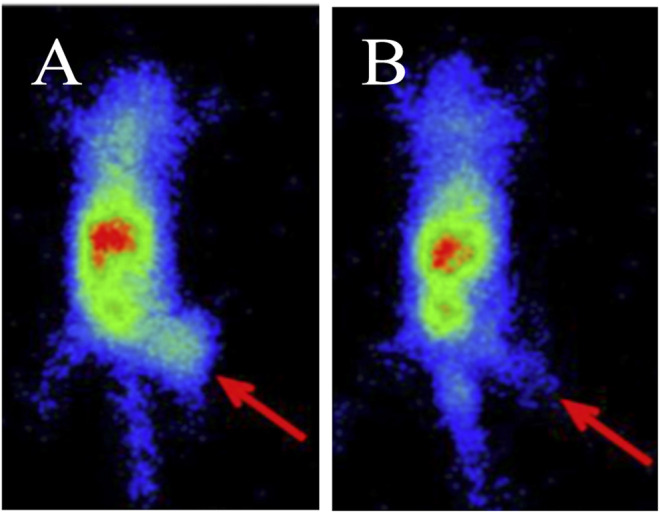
SPECT Imaging of MDM2 expression in MCF-7 xenografts using ^99m^Tc-HYNIC-antisense (A) and mismatch (B) probes at 4 h post-injection. Tumors are indicated by red arrows (Fu et al., 2010). The general structure of ^99m^Tc-HYNIC-antisense was shown in Figure 2A.
